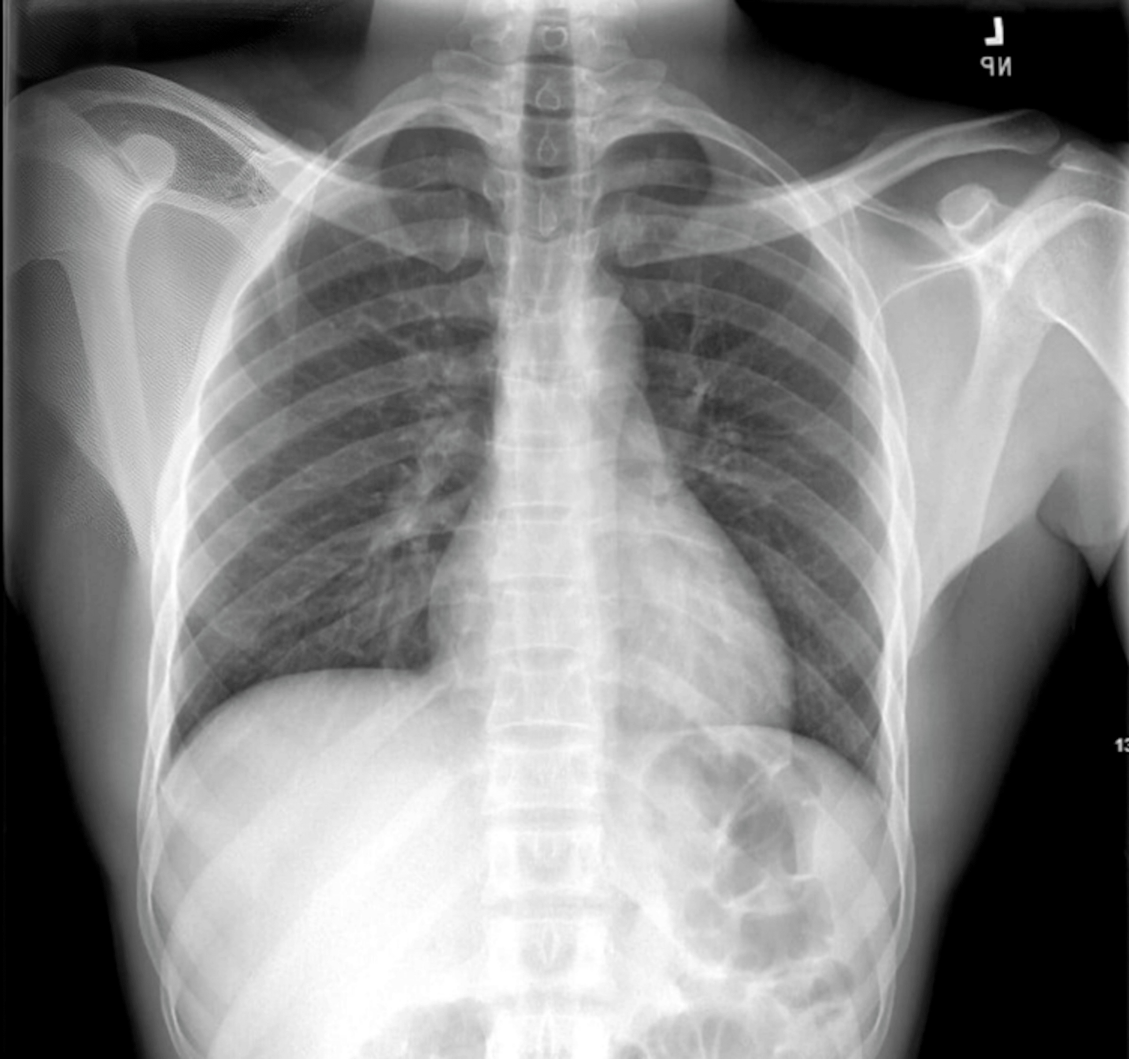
Chest X-ray showing no evidence of consolidation or atelectasis indicative of infection
